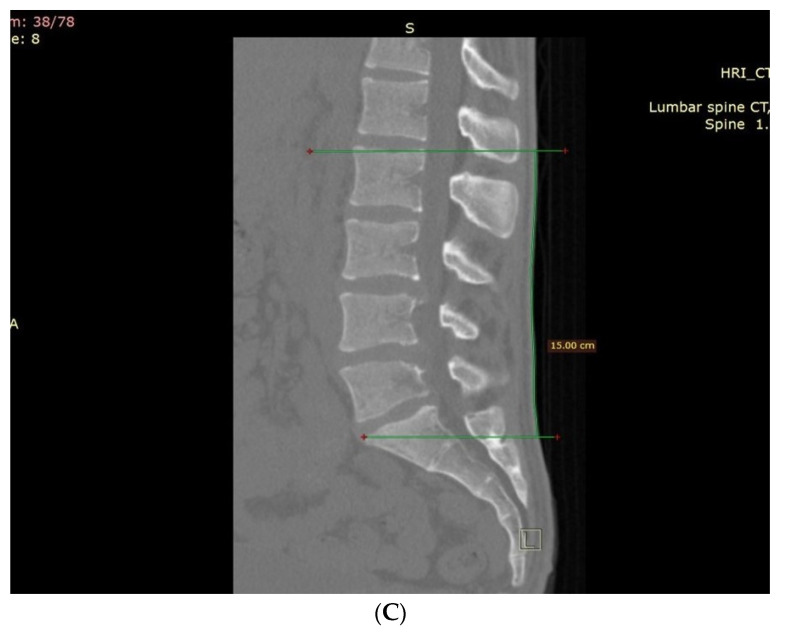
MMST Measurement. (A) MMST PSIS LANDMARK on CT axial–sagittal views. (B) MMST PSIS Qaudal starting point. MMST was measured by marking the most prominent point of the Posterior Superior Iliac Spine (PSIS), as measured by CT (Marked arrows). On that level, scrolling back to a midline sagittal plane, a parallel line was drawn to the skin surface as described previously. (C) MMST complete CT measurement. From that point, a 15 cm skin surface line was drawn cranially. The caudal and cephalad spinal levels were measured as described before.
